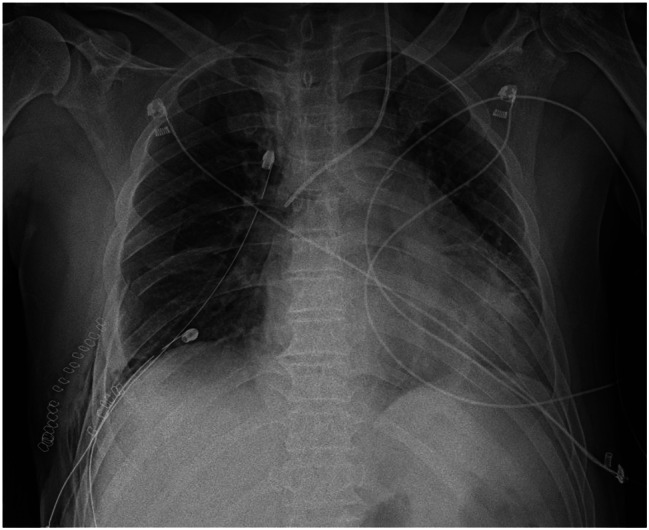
Plain chest X-ray findings: Radiolucency of the right lower lobe was markedly improved
